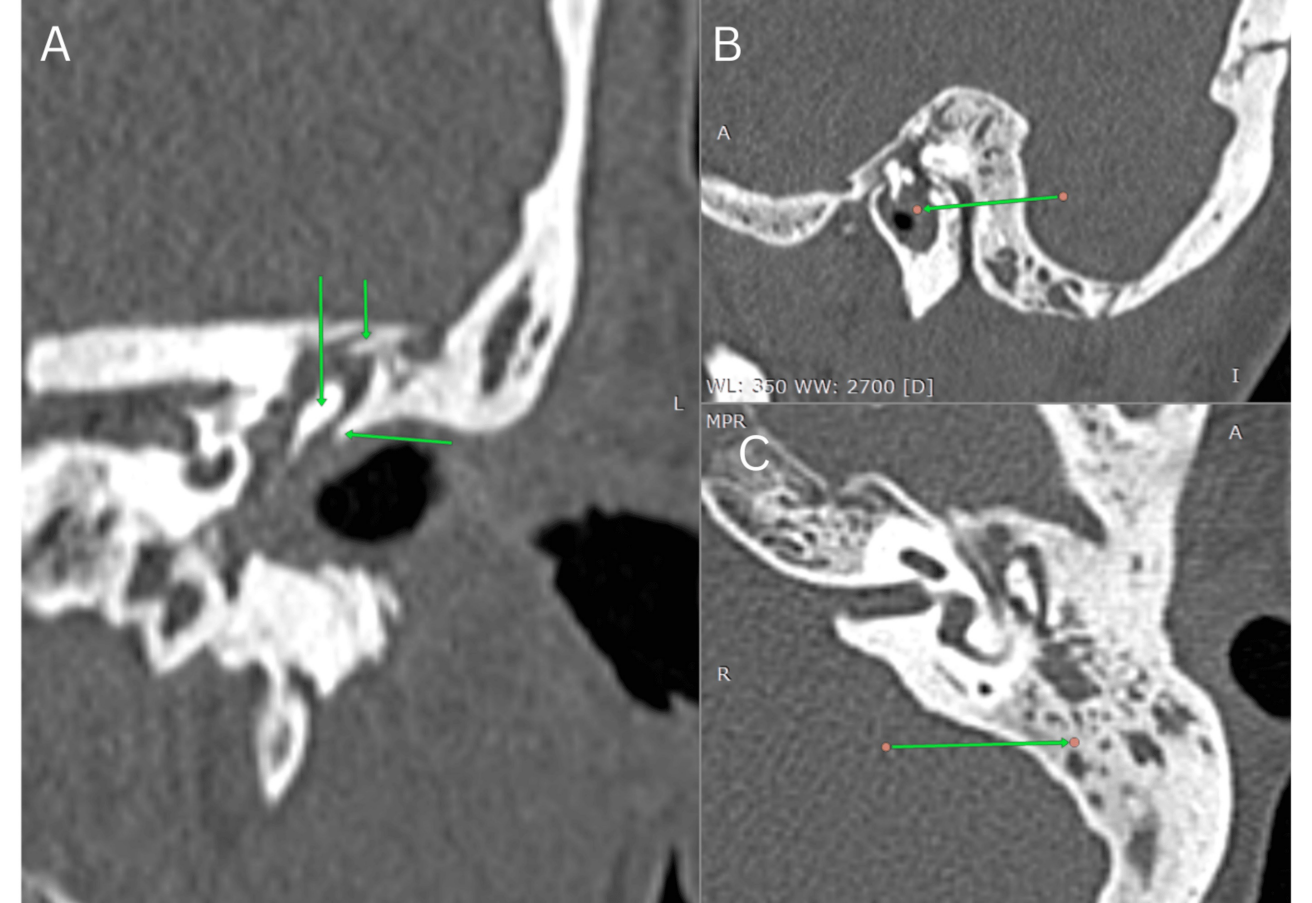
Follow-up high-resolution computed tomography of the temporal bone (May 2025): interval improvement after medical therapy (A) Sagittal/oblique view: marked reduction of soft tissue opacification in the left middle ear and mastoid, showing re-aeration (green arrows). (B) Axial view: near-complete clearing of mastoid air cells: minimal residual soft tissue. (C) Coronal view: reduced middle ear and mastoid effusion, along with enhanced visibility of the tympanic cavity and mastoid antrum.
